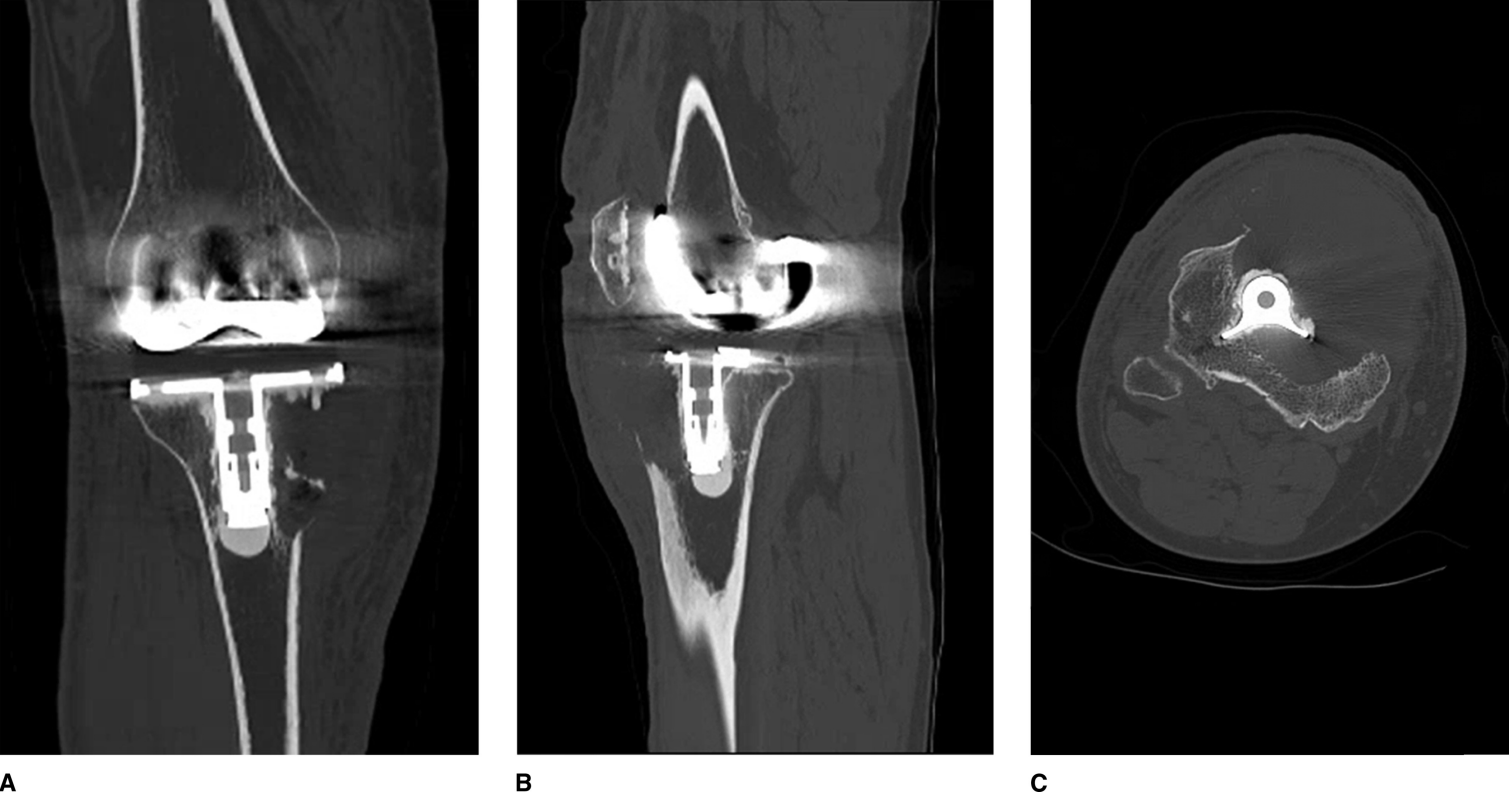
Coronal (A), sagittal (B), and axial (C) images of preoperative CT of the right knee demonstrating a large expansile destructive lytic lesion of the proximal tibia abutting the tibial component of the total knee arthroplasty.
